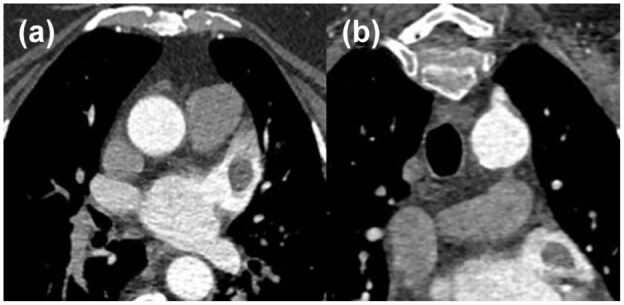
Positive CTA imaging demonstrating free-floating LAA thrombus on axial (a) and coronal (b) sequences.
